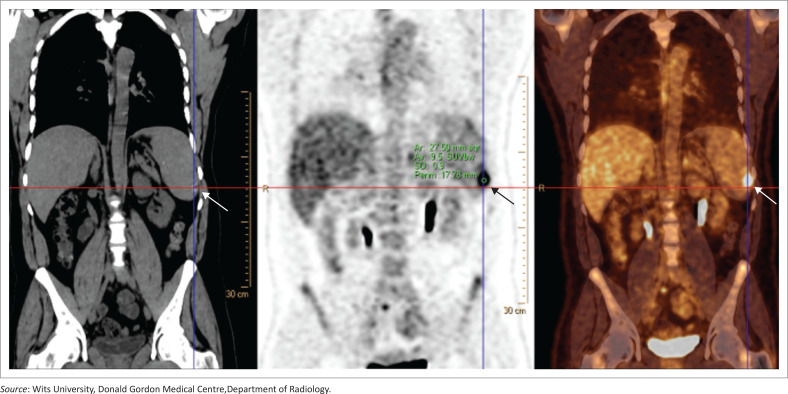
Peritoneal metastatic deposit mimicking a splenic lesion in a 37-year-old patient with a history of rectal adenocarcinoma. Coronal FDG PET demonstrates a peripheral stippled calcified focus with intense FDG uptake (SUV 9.5). Multiplanar reformats (not shown) confirmed that the abnormality was not intraparenchymal but instead located along the capsular surface of the spleen.
